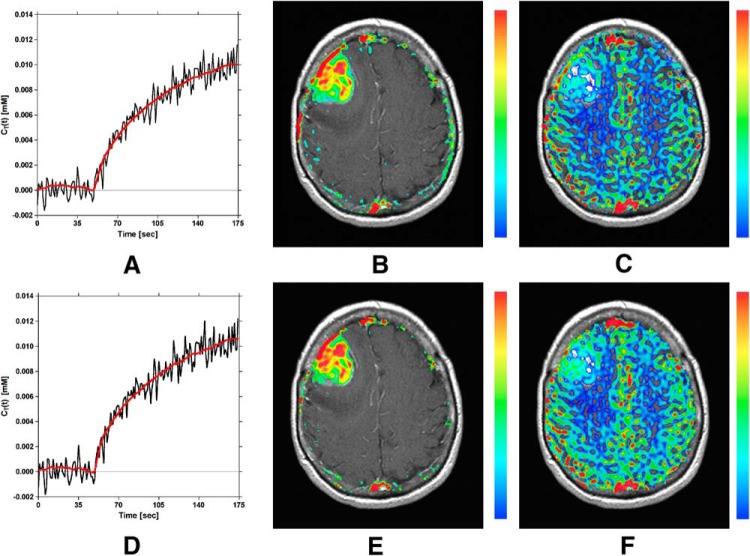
Demonstration of the correction of DCE-MRI data using the proposed SPICE method. Top row: Linear least squares Patlak model fit (red line) to tissue concentration–time curve (A), and corresponding estimates of K^trans^ (B) and v_p_ (C). The concentration–time curve in (A) was constructed using a single echo signal time course analogous to conventional DCE analysis. Second row: Linear least squares Patlak model fit (red line) to tissue concentration–time curve (D), and corresponding estimates of K^trans^ (E) and v_p_ (F). The concentration–time curve in (D) was constructed using a dual-echo corrected signal time course, which facilitated correction for *T*_2_*(t) effects.
